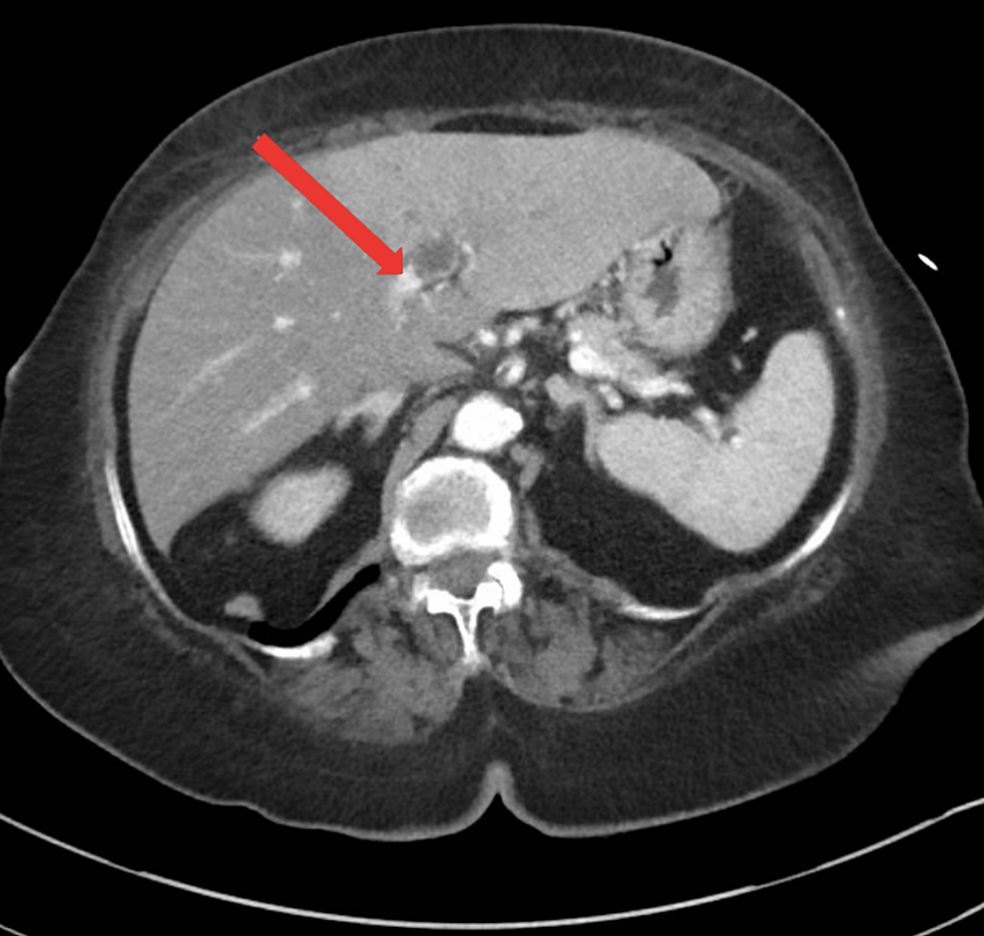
Initial computed tomography of the abdomen showing portal vein thrombosis
